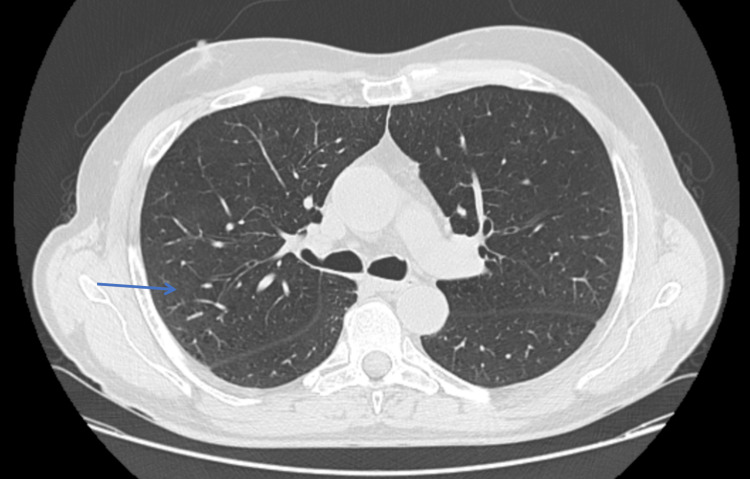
Chest CT in the axial plane showing the improvement of the diffuse tree-in-bud opacities in the area highlighted by the blue arrow.
